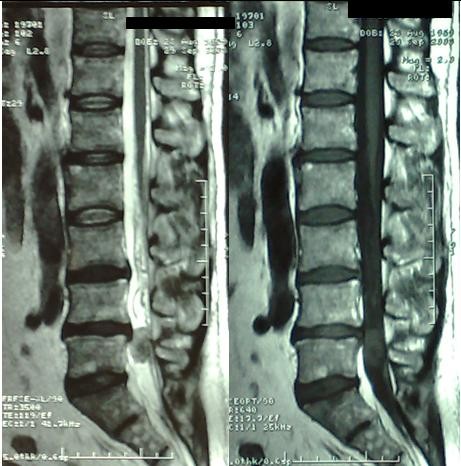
Preoperative MRI. Sagittal T2 and T1 weighted images with Gd-DTPA.
